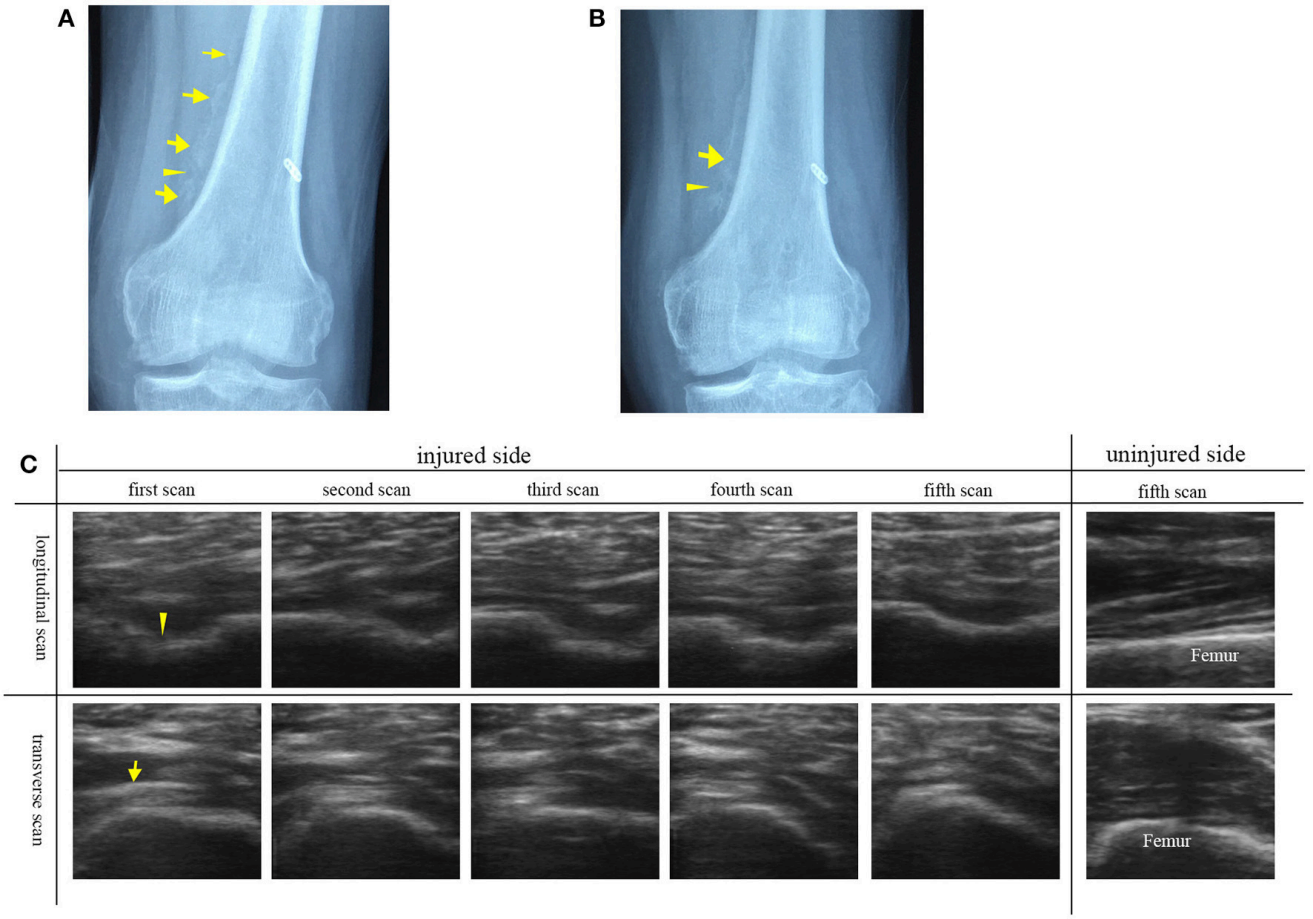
Plain radiographs and ultrasound images of left knee joint of participant No. 8 with HO in the immature bone formation stage. (A) The plain radiograph was taken as the participant was in hospital. Discontinuous (arrowhead), faint and poorly demarcated (arrows) calcification was found. (B) The second plain radiograph was taken ~1 month after the first plain radiograph. Continuous (arrowhead) and distinguishable (arrow) HO was found. (C) A series of transverse and longitudinal ultrasound images of the injured joint in comparison with the uninjured side. HO is visualized during its development. The unconnected HO tissues (arrowhead) correspond to the gap between two calcified HO tissues. On the fifth ultrasound images, the HO tissues connect smoothly to develop into the mature HO tissues. As the echogenicity and homogenesis of HO increased, the profile of the femur become blurred (arrow).
